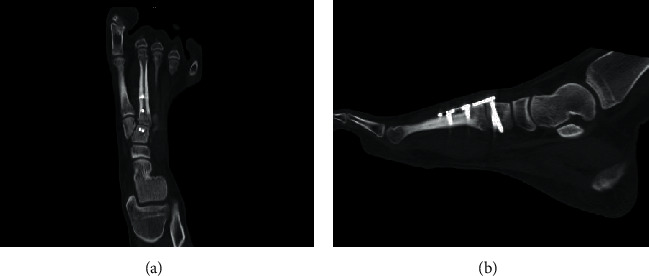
At 12 weeks after the operation, CT demonstrated complete bone union at the fracture site on (a) coronal and (b) sagittal views.
